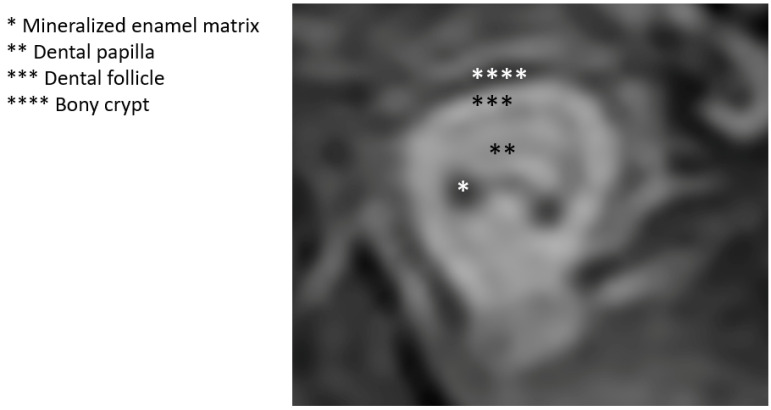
Post-appositional tooth germ anatomy shown on T2-weighted coronal MRI. Note the hypointense (dark) signal corresponding to hypocellular mineralized tissues (bony crypt and enamel).
